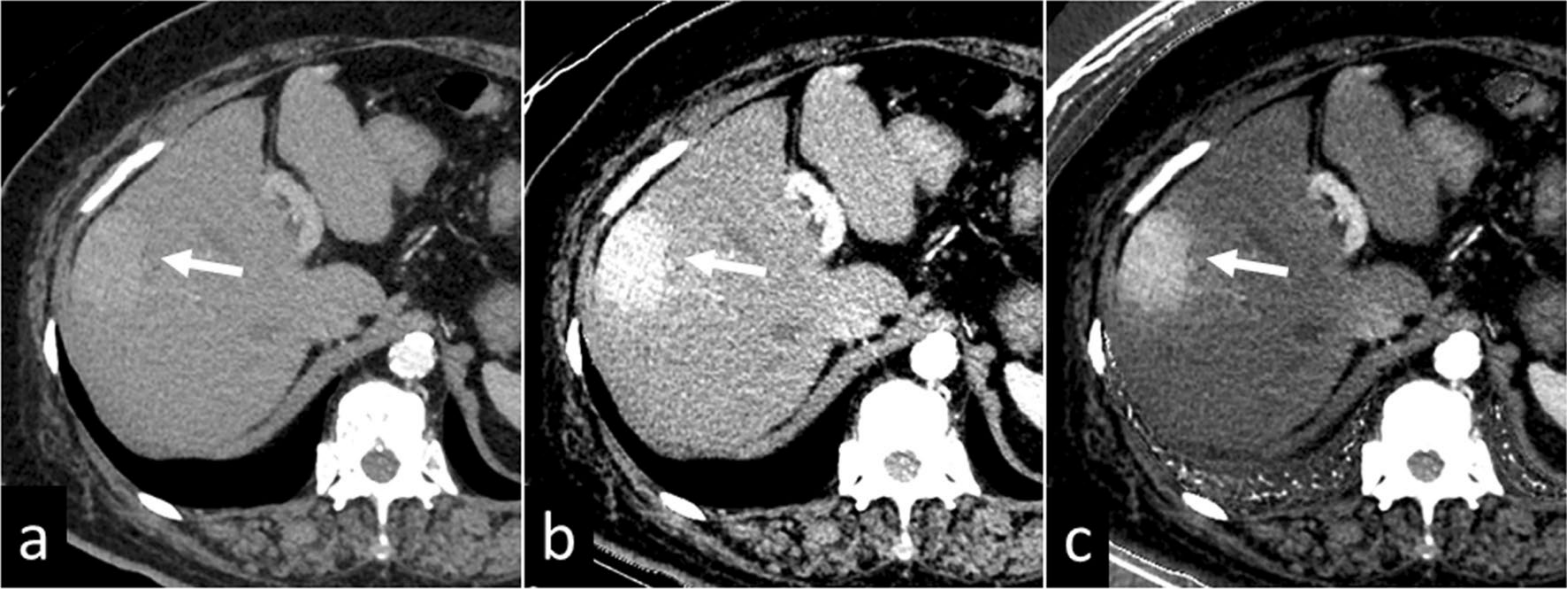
A 64-year-old woman with hepatocellular carcinoma. CT images during the arterial phase were obtained with a low contrast material dose (220 mgI/kg) due to renal insufficiency (eGFR, 21 ml min^–1^ 1.73 m^–2^). Visualization of the liver lesion is insufficient on the virtual monochromatic 70 keV image (a), whereas it is clearly detected on the monochromatic 40 keV image (b), and the iodine map (c)
